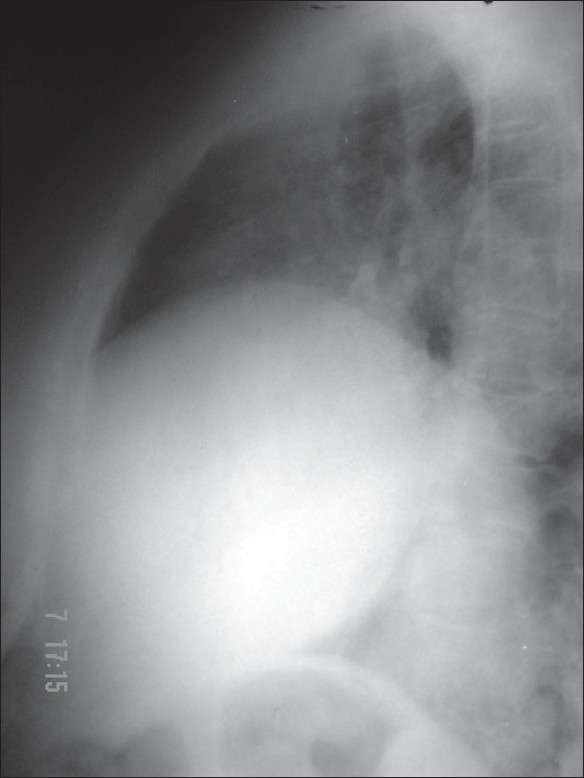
Chest X-ray right lateral view showing eventration of right dome of diaphragm
